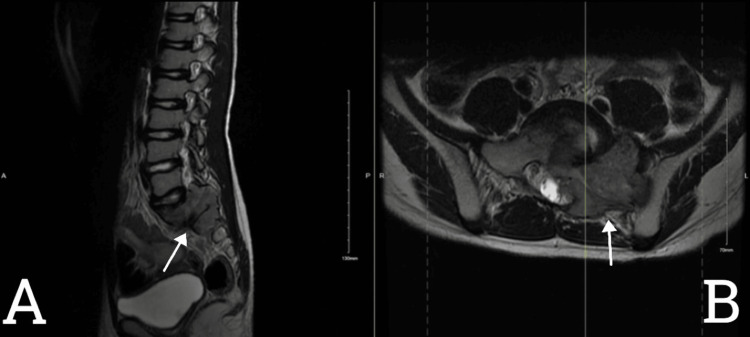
The MRI lumbosacral T2WI sagittal view (A) and axial view (B) An extensive L5–S2 lesion causing destruction of the left side of sacral ala and posterior elements of S1–S2 can be seen in both sagittal and axial views. It extends to the central canal, S1–S2 neural foreman, and left sacroiliac articulation. There is mild left paravertebral muscular extension and atrophy of the left pisiform muscle, likely related to chronic nerve compression by the mass. There are also signal changes manifested by T2WI hyperintensities and enhancement, for tumor involvement.
